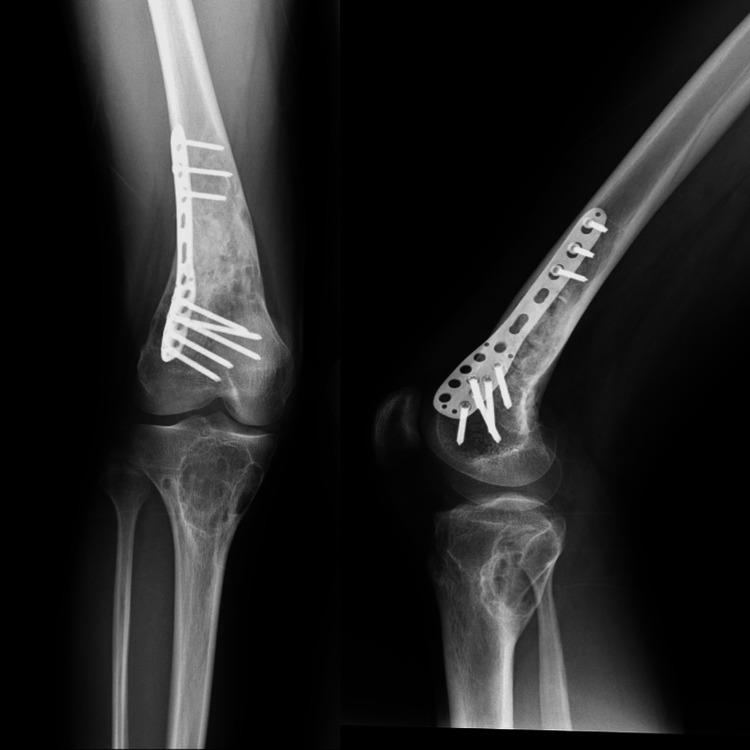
Postoperative radiograph of the patient taken at six months
